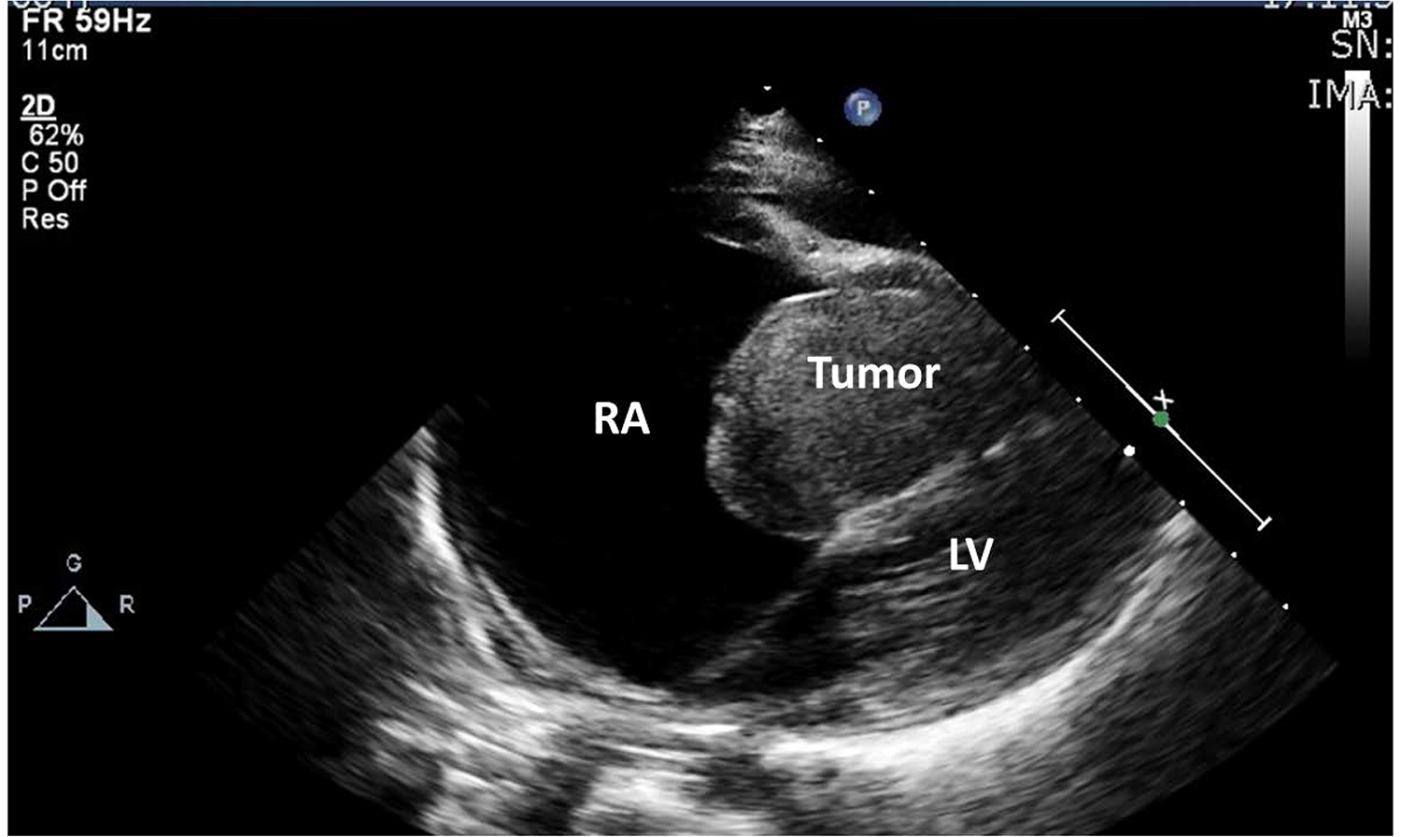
Echocardiographic image of large dilated right atrium with large mass in the right ventricular cavity towards the right atrium; no discernable tricuspid valve.
